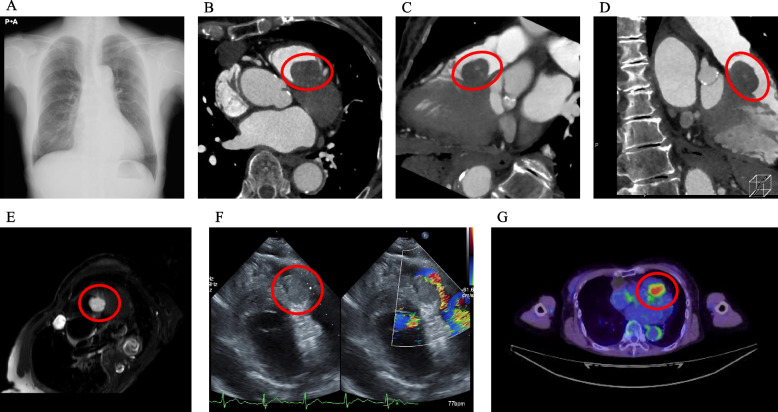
Preoperative findings. A Chest X-ray. B, C, D Computed tomography. E Magnetic resonance imaging (T2W). F Transthoracic echocardiography. G FDG-PET/CT (a tumor shown as a red circle)
